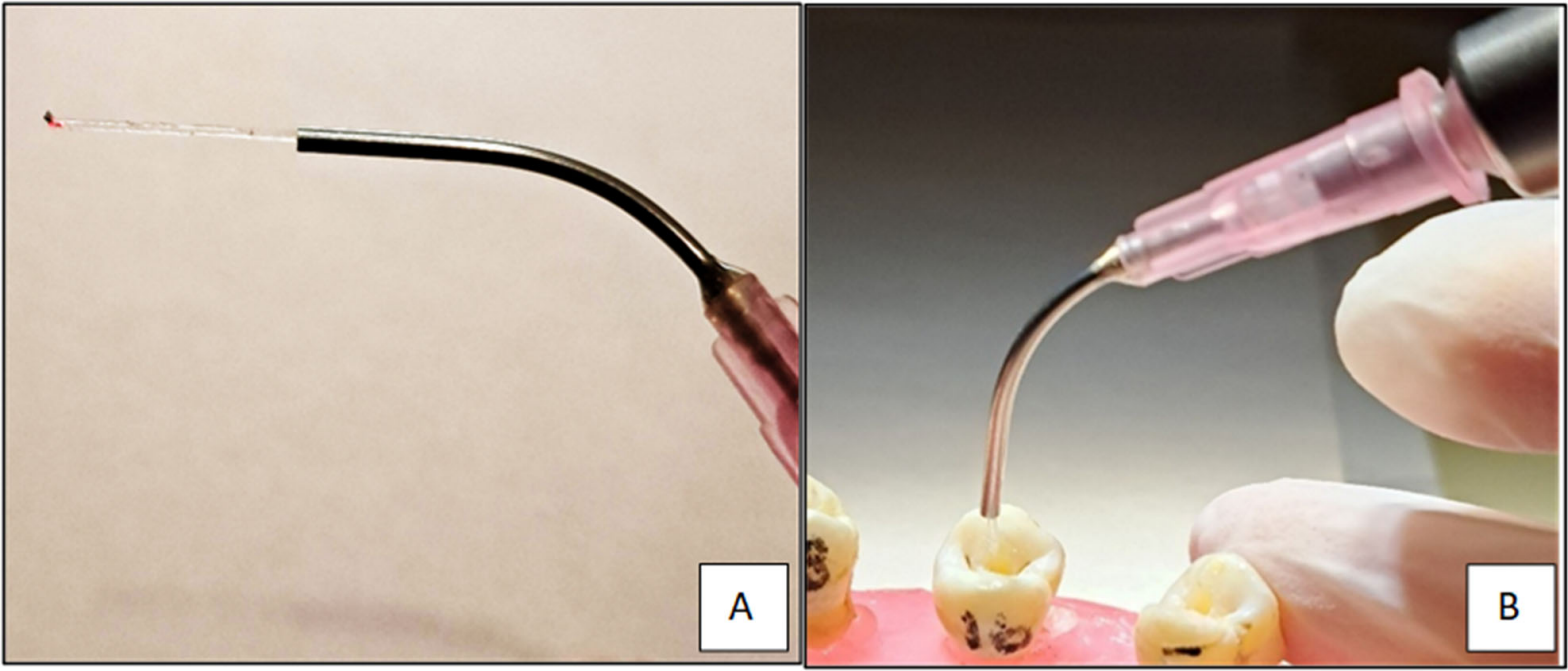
Application of Diode laser. A Diode laser 200 µm x 15 mm fiber-optic cable; B Cable inserted along the 1 mm from the radiographic apex of the root canal.
